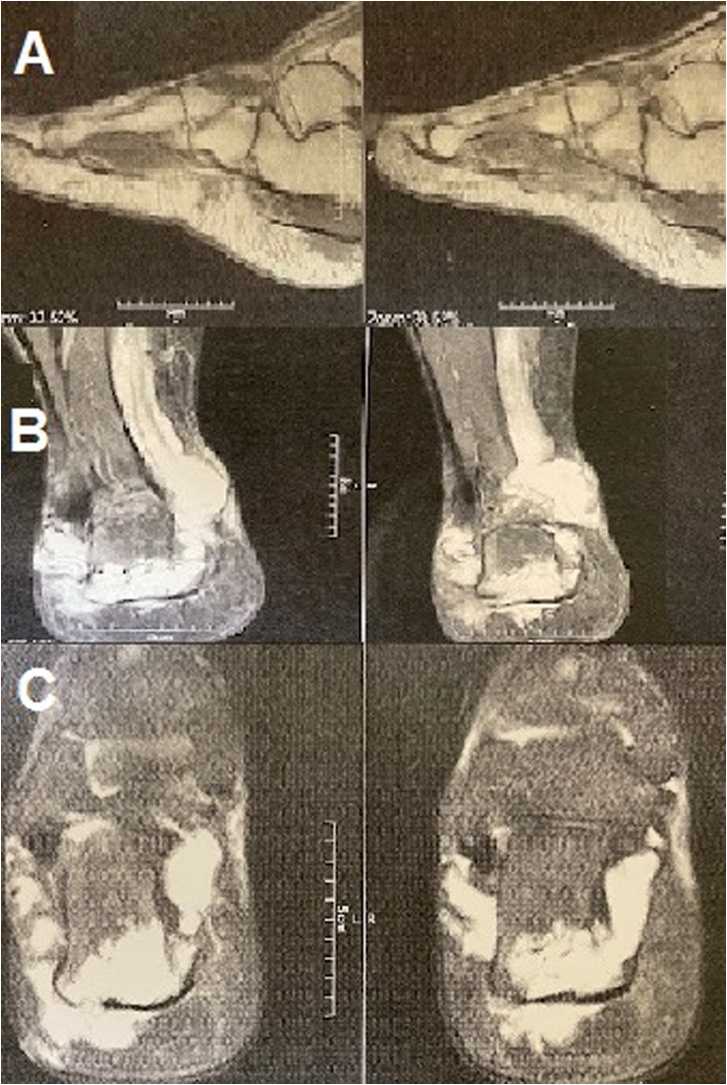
T1- and T2-weighted MRI in sagittal (A, B) and axial (c) planes demonstrating a large soft tissue mass superficial to the deep plantar fascial plane and embedded within the subcutaneous and deep dermis tissue. The lesion has a low signal intensity on T1weighted images (A) and heterogeneous signal intensity on T2-weighted images (B, C).
